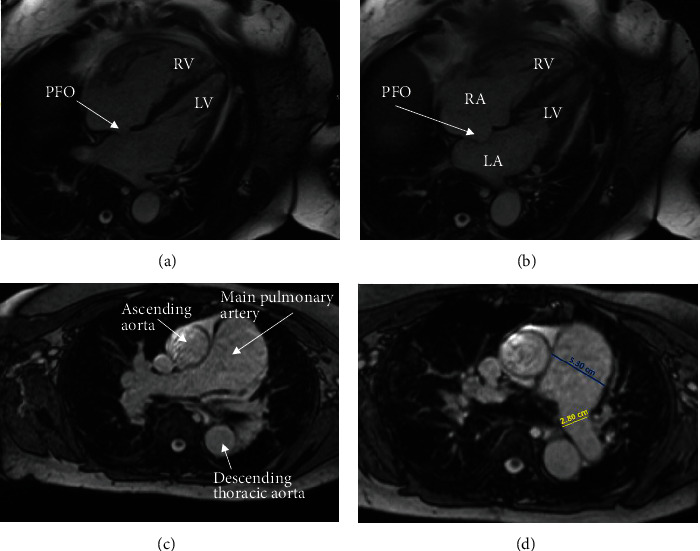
Cardiac MRI revealing the patent foramen ovale (PFO). (a, b) The PFO (white arrow) and interatrial aneurysm. (c, d) The dilated main pulmonary artery. In (d), the blue line denotes the main pulmonary artery (5.30 cm), and the yellow line denotes the left pulmonary artery (2.80 cm). LA, left atrium; LV, left ventricle; RA, right atrium; RV, right ventricle.
